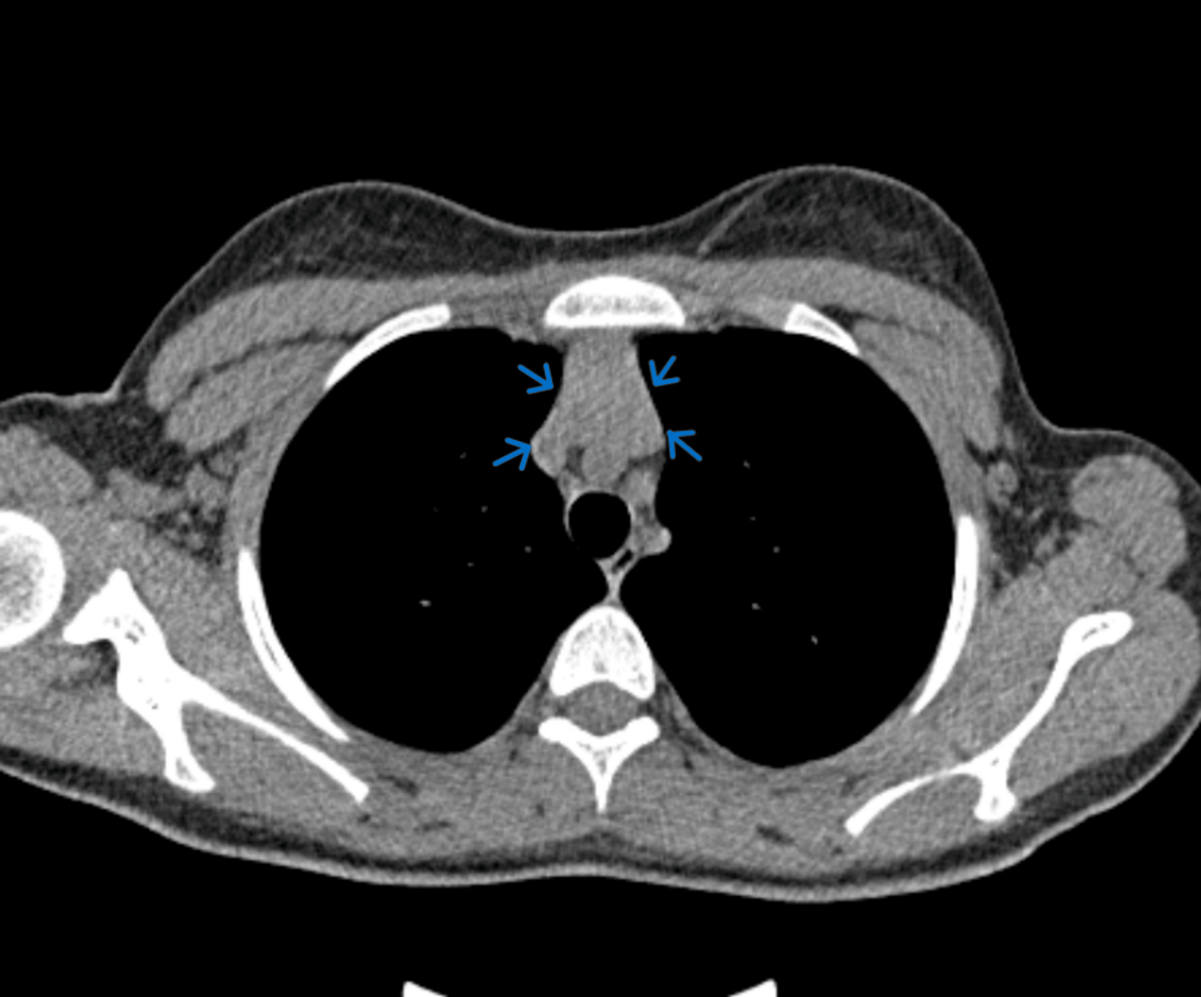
The chest CT scan in thymic hyperplasia CT: computed tomography
